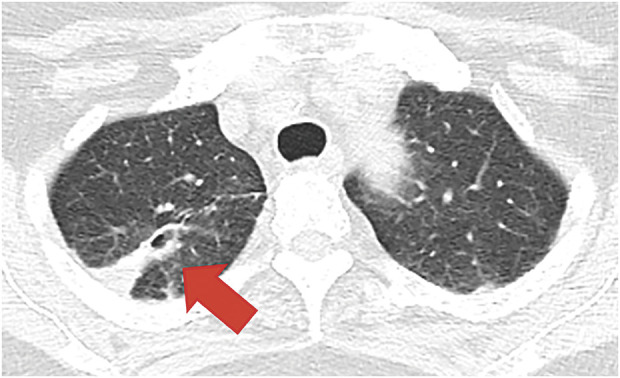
Chest computed tomography 26 months after the first treatment. The mass in the right upper lobe of the lung disappeared (red arrow); the image shows atelectasis.
